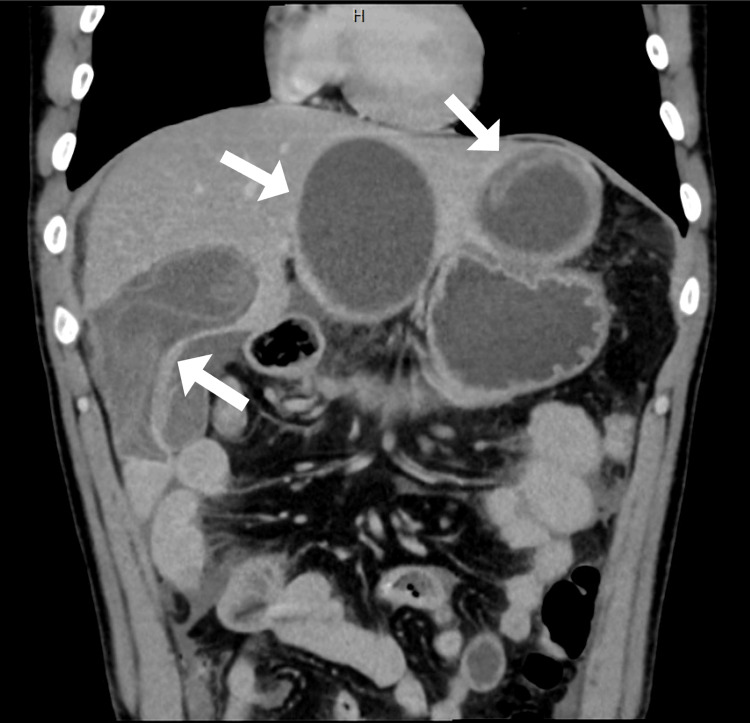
Coronal CT image demonstrating multiple hydatid cysts within the liver
